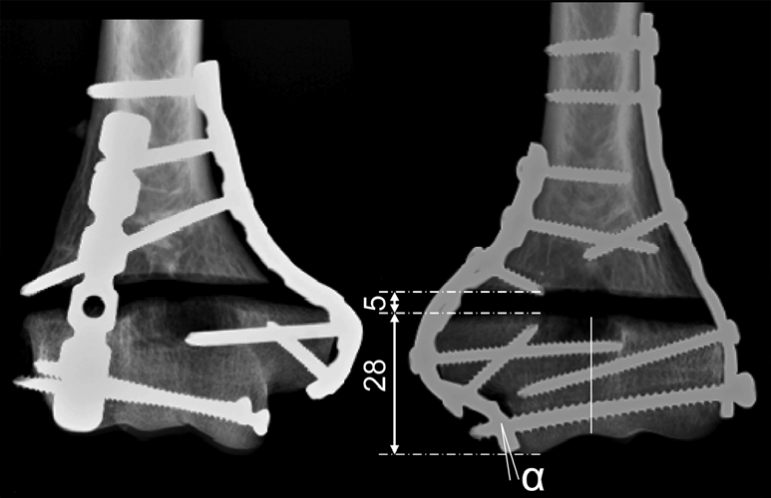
Anteroposterior radiographs of an operated bone pair. Left: LCP construct with 90° plate arrangement. Right: "Frame" technique with interconnection between both plates parallel to the joint surface for establishment of a "U"-shape configuration. The interconnection screw engages with the reconstruction plate. α indicates an oblique orientation of the plate, which further promotes the anchorage of the screw. Numbers are given in mm.
